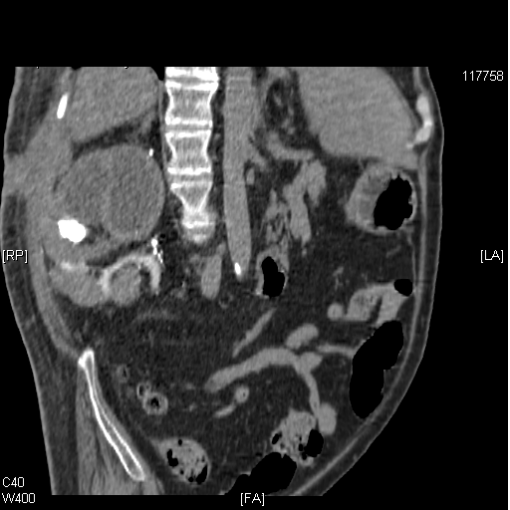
Sagittal reconstruction of the CT scan of the abdomen, performed on 5 March 2007. Communication between the cyst in the right kidney and the exterior through a defect in the anterior abdominal wall musculature is revealed.
